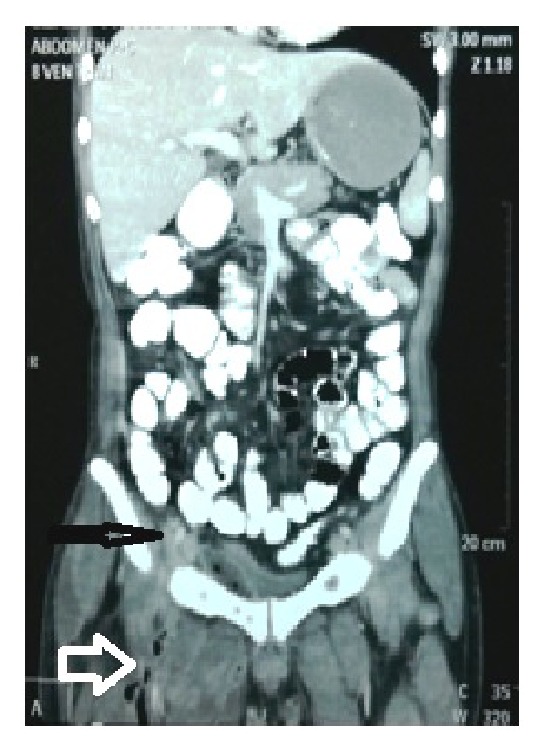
Contrast enhanced CT showing contrast leak (thin black arrow) from the base of the appendix and air specs in soft tissue of the thigh (bold white arrow).
